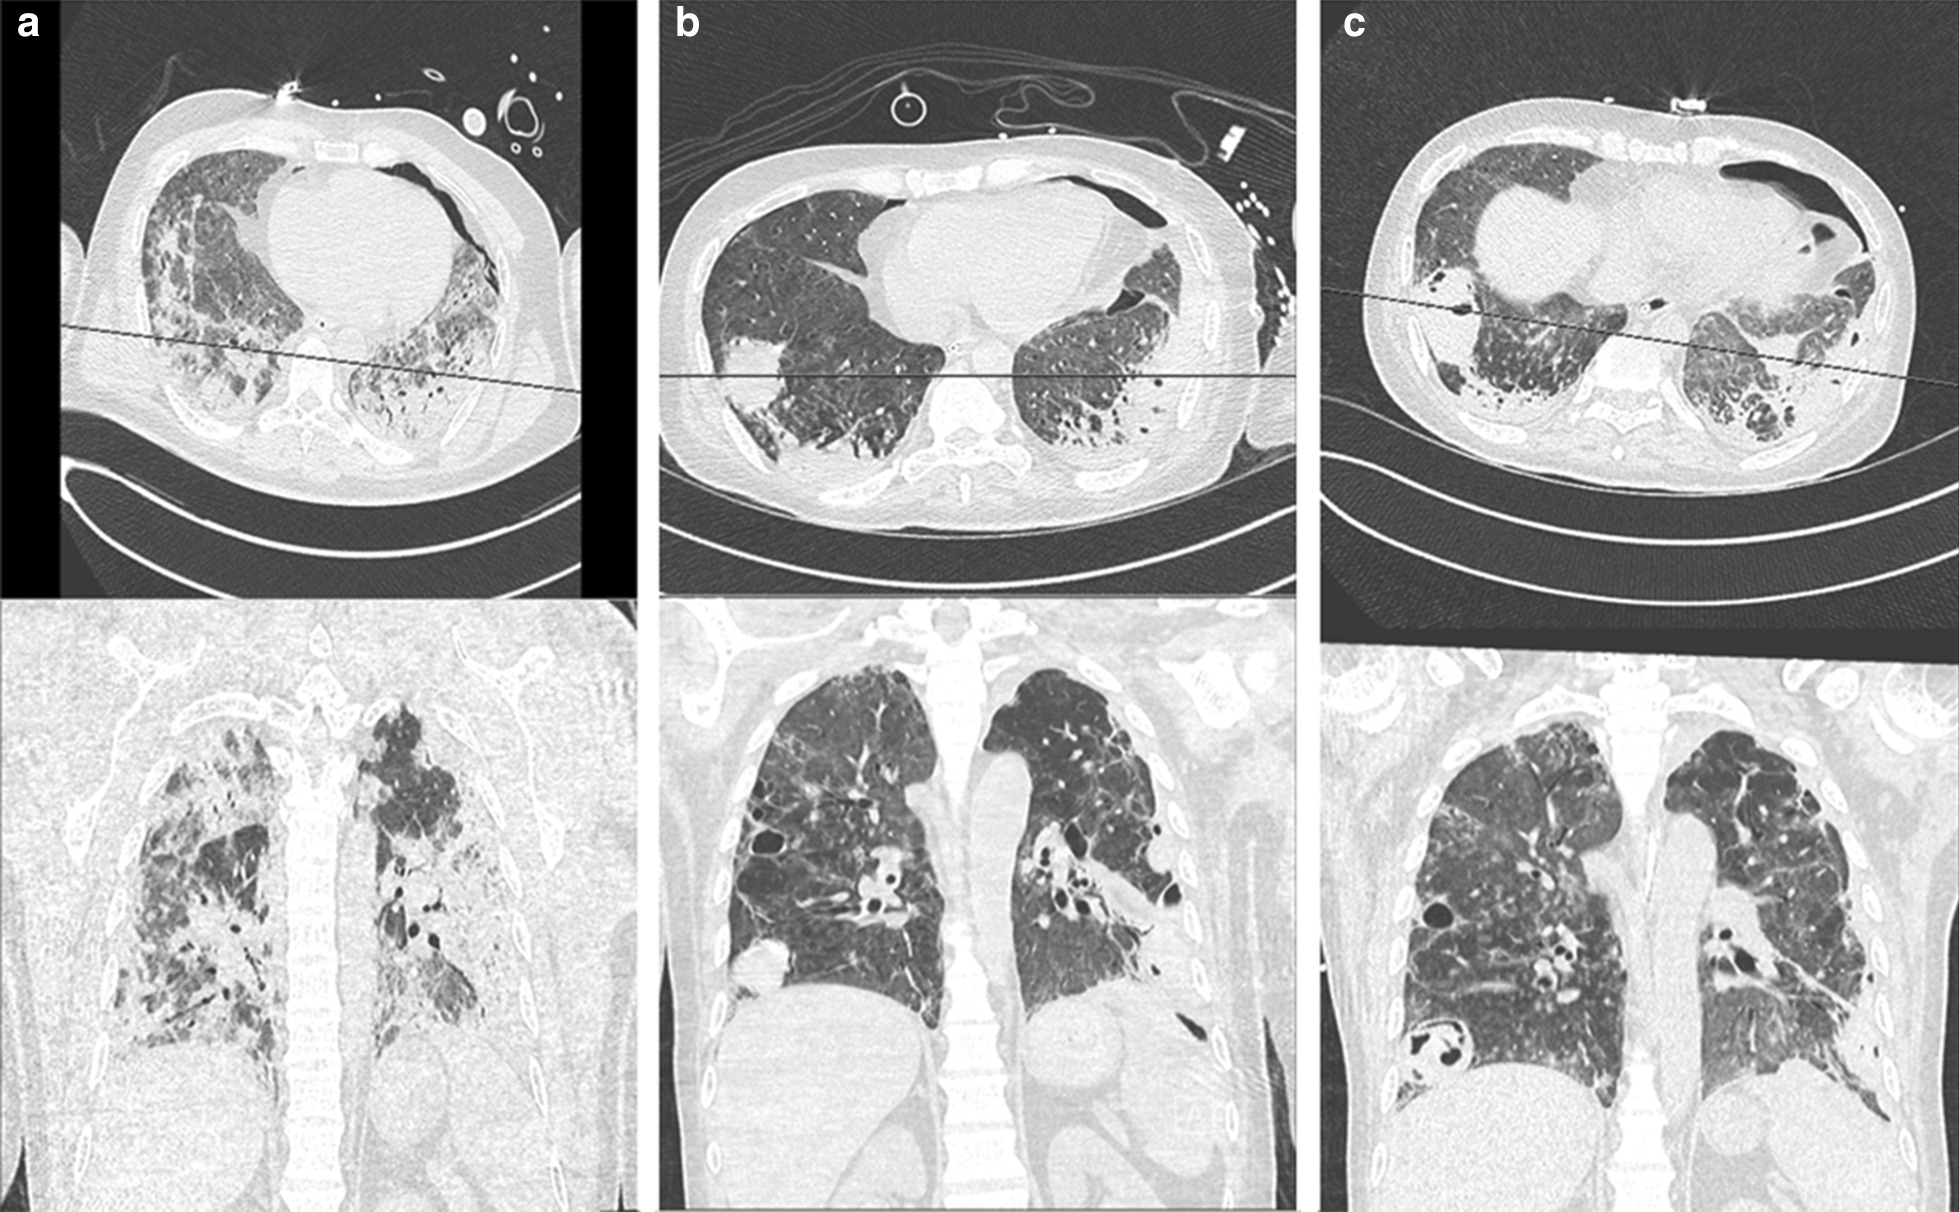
Axial and coronal CT images at form patient 9 on a 14th May 2020, b 8th June 2020, and c 18th June 2020
